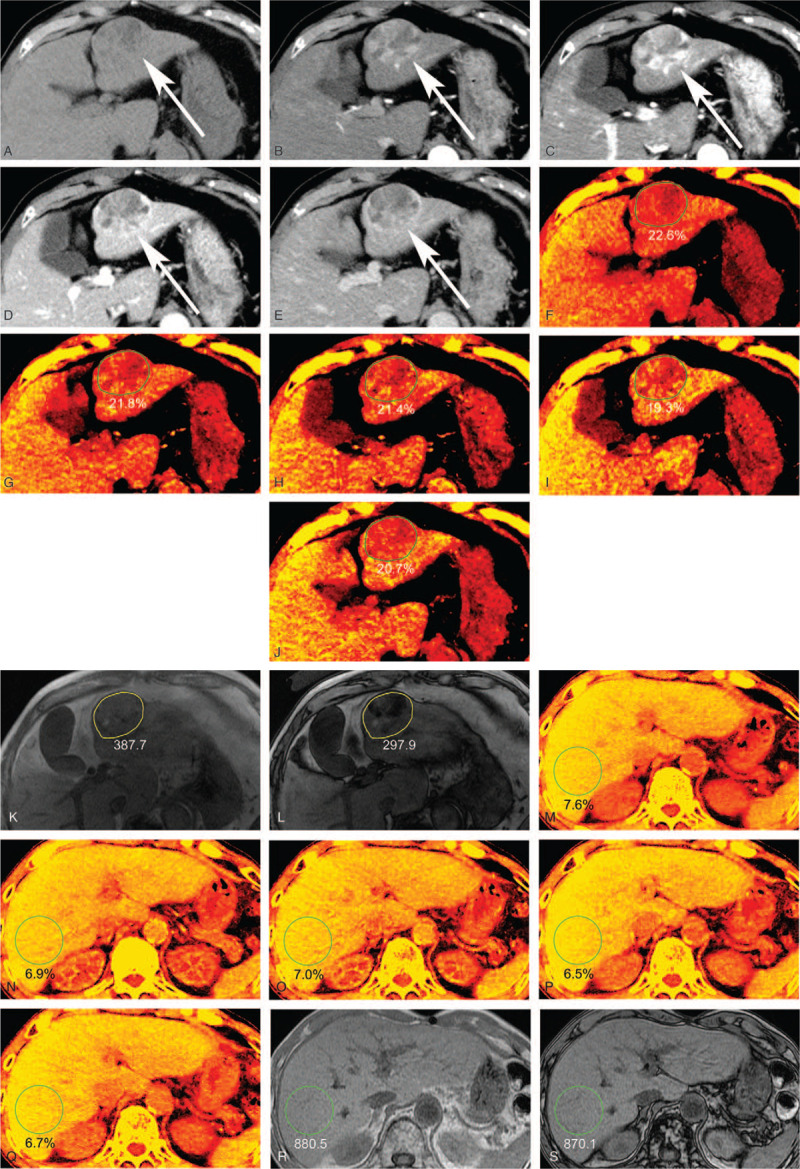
A 79-year-old male with HCC with fat component. Non- and four-CE CT (A–E) and FVF maps by using the MMD algorithm in each phase (F–J) and in-phase and opposed-phase images (K, L). (A) Non-CE CT shows a 5-cm-sized hepatic mass with a hypodense area in segment 3 (arrow). (B, C) On AP1 and AP2, the tumor shows nonrim arterial hyperenhancement (arrow). (D, E) On PVP and EP, the tumor shows nonperipheral “washout” (arrow). Enhancing capsule appearance is also seen in EP. The tumor is categorized as LR-5 (definitely HCC) by using the LI-RADS version 2018. (F) In the FVF map, FVF (%) of the tumor shows (F) 22.6% in non-CE, (G) 21.8% in AP1, (H) 21.4% in AP2, (I) 19.3% in PVP, and (J) 20.7% in EP by placing freehand ROIs (green circle). (K) Signal intensities of the tumor are 387.7 on in-phase image and (L) 297.9 on opposed-phase image by placing freehand ROIs (yellow circle). FF_CSI_ yields 11.6%. Surgical pathological findings confirmed “moderately differentiated HCC.” A 74-year-old male with HCC. FVF maps by using the MMD algorithm in each phase (M–Q) and in-phase and opposed-phase images (R, S). Circle ROI was placed on the right robe homogeneous area. In the FVF map, FVF (%) of the liver parenchyma shows (M) 7.6% in non-CE, (N) 6.9% in AP1, (O) 7.0% in AP2, (P) 6.5% in PVP, and (Q) 6.7% in EP by placing freehand ROIs (green circle). (R) Signal intensities of the liver are 880.5 on in-phase image and (S) 870.1 on opposed-phase image by placing circle ROIs (yellow circle). FF_CSI_ yields 0.6%. AP1 = early arterial phase, AP2 = late arterial phase, EP = equilibrium phase, FF_CSI_ = fat fraction calculated from chemical shift imaging, four-CE = four-phases contrast-enhanced, FVF = fat volume fraction, HCC = hepatocellular carcinoma, MMD = multimaterial decomposition, non-CE = non-contrast-enhanced, PVP = portal venous phase.
